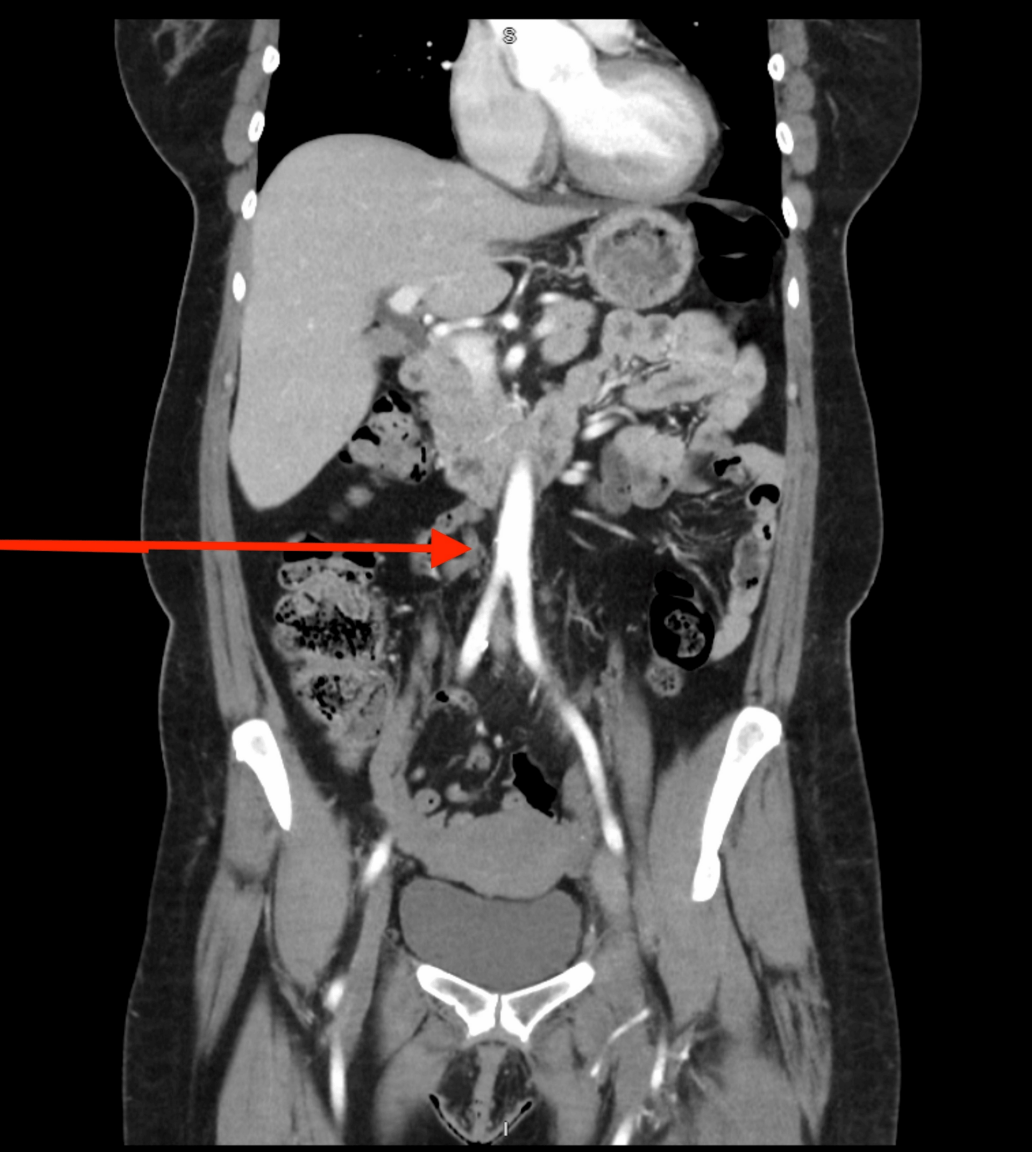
CT abdomen and pelvis showing absence of inferior vena cava in the abdomen (red arrow)
